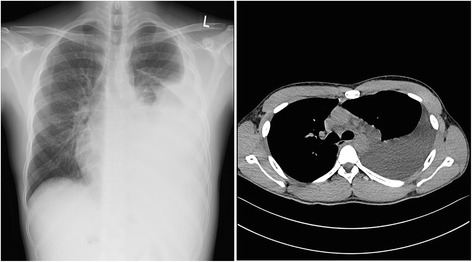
Chest PA and chest CT showing left-sided pleural effusion
